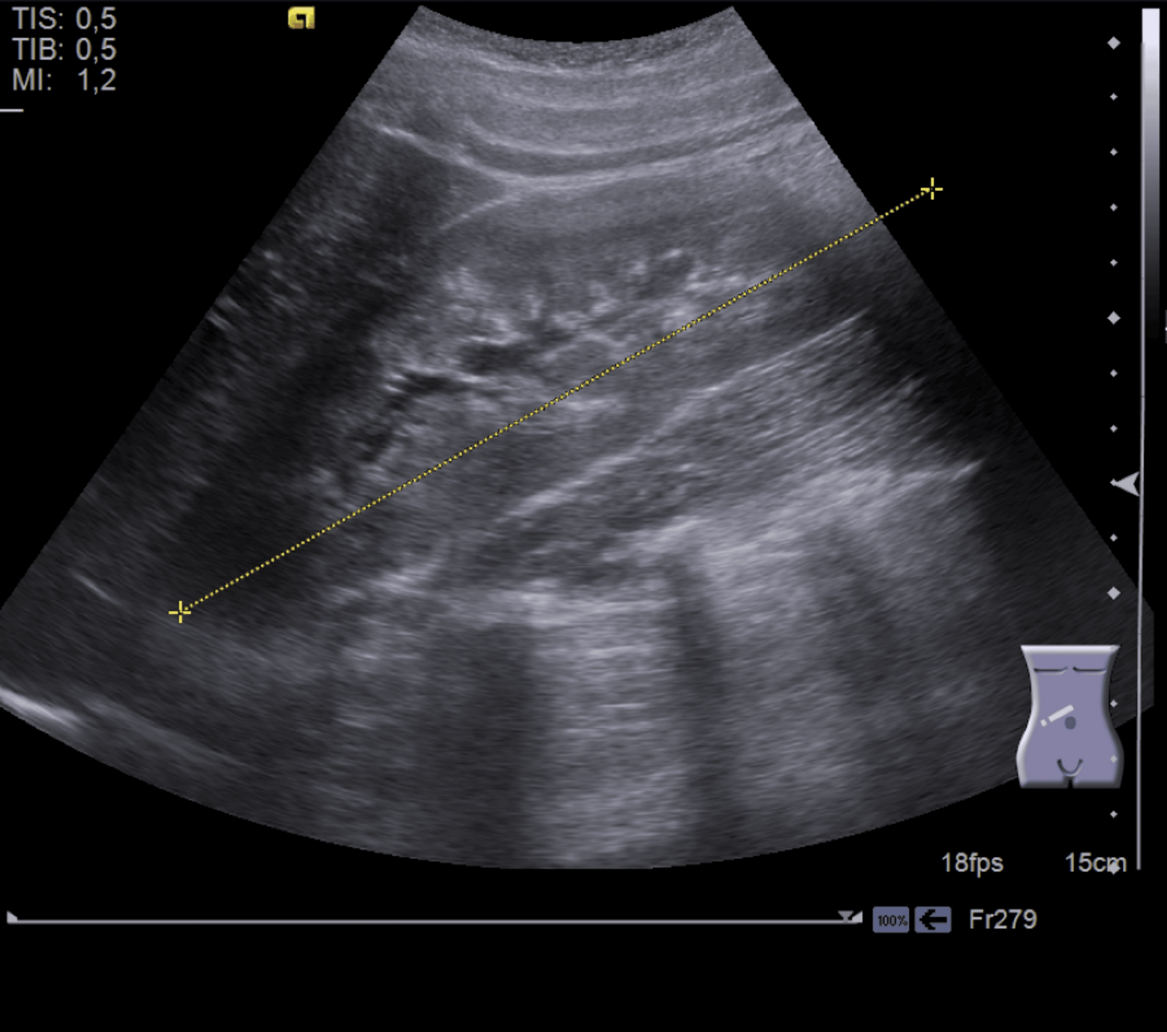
Longitudinal pelvic ultrasound Right kidney in normal topography, with compensatory-type morphology and increased volume, longitudinal diameter of 150 mm, regular contour, normal parenchyma-sinus differentiation, and preserved parenchymal thickness TIS: thermal index for soft tissue; TIB: thermal index for bone; MI: mechanical index
